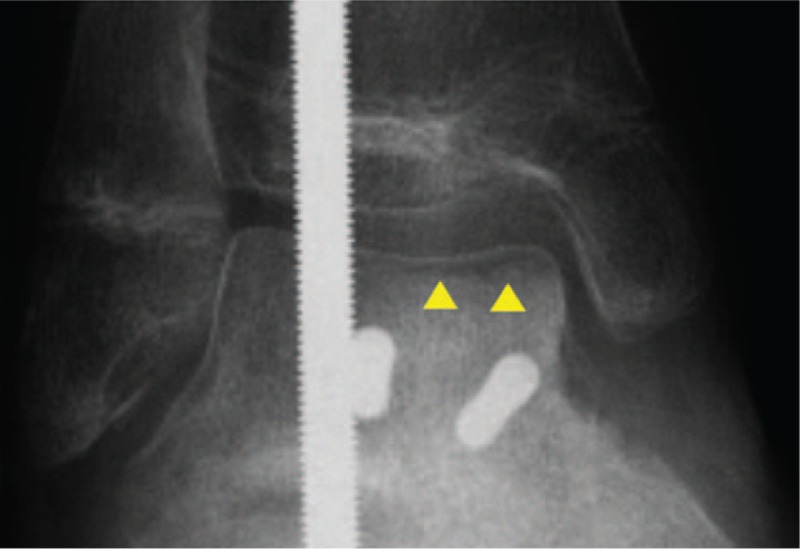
Anterior-posterior radiograph showing subchondral radiolucent band in the talus dome (arrow head).
